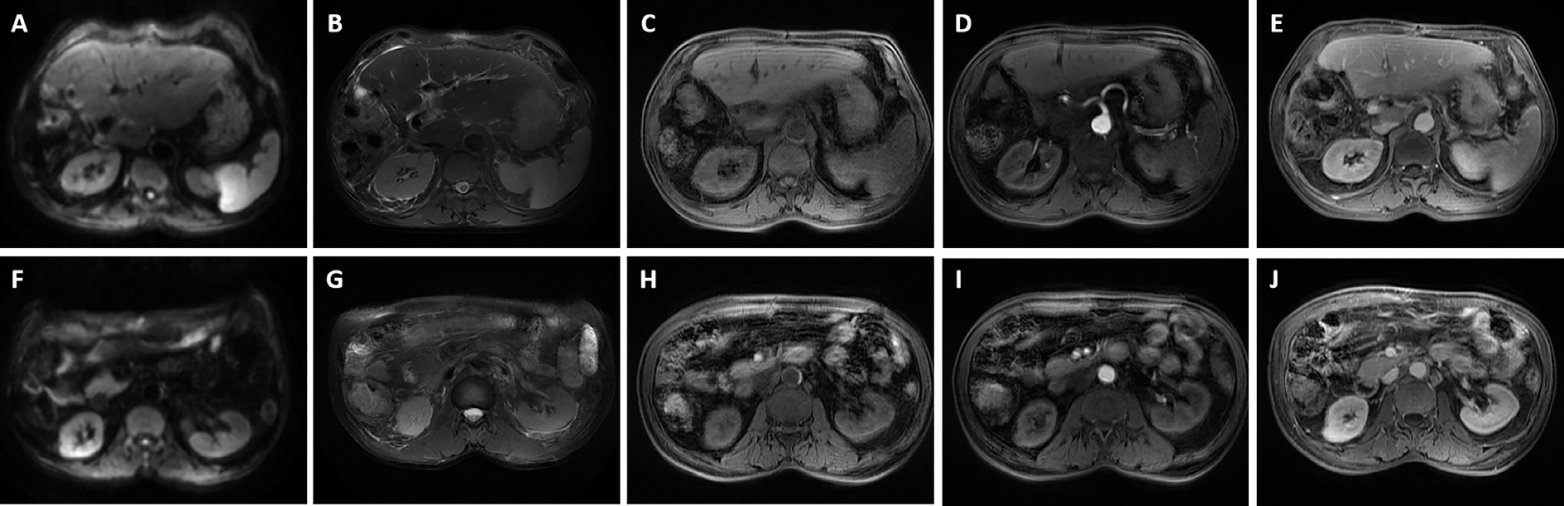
Magnetic resonance imaging was performed for the patient 1 month after surgery. It revealed postoperative changes of liver and gallbladder, a little exudation and effusion in the operative area. (A, F) Diffusion-weighted imaging (DWI); (B, G) T2-weighted imaging; (C, H) T1-weighted imaging; (D, I) The arterial phase; (E, J) The delayed phase.
